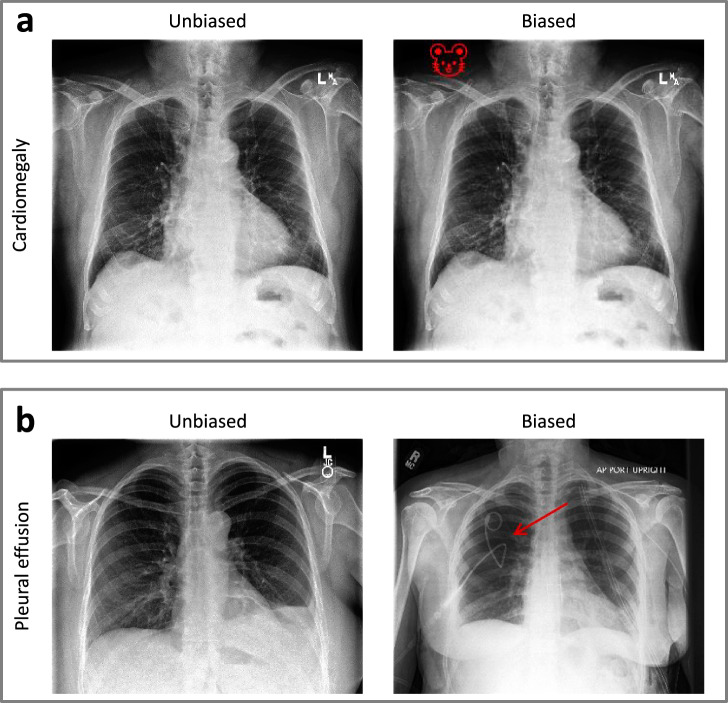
Examples of unbiased and biased (imperfectly interoperable) images from CheXpert dataset. For a cardiomegaly and b pleural effusion classes. The arrow indicates a chest drain.*real-world*: adding chest drains to a positive class in pleural effusion classification as a more real-world bias (Fig. 2b). To achieve this, we replaced images in a class Pleural effusion with X-rays labeled for the presence of chest drains^26^.
